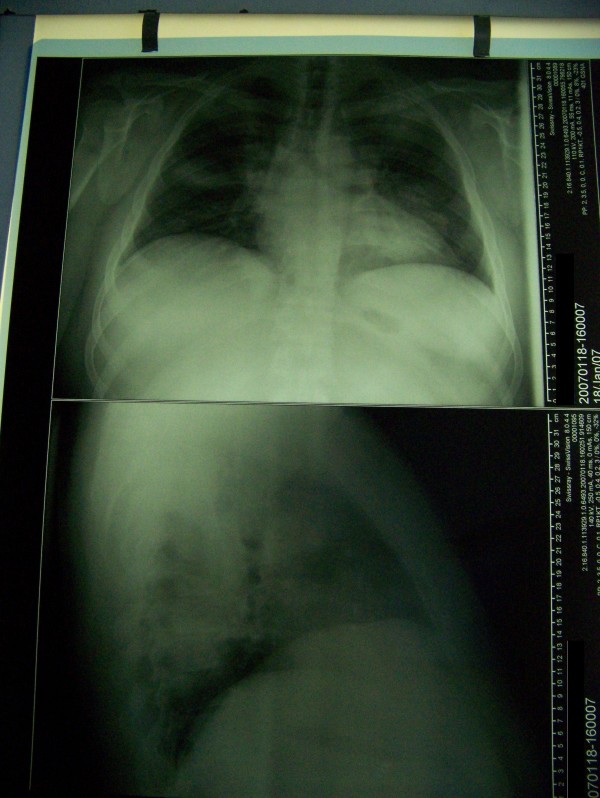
Chest x-ray shows bilateral pneumonia.
